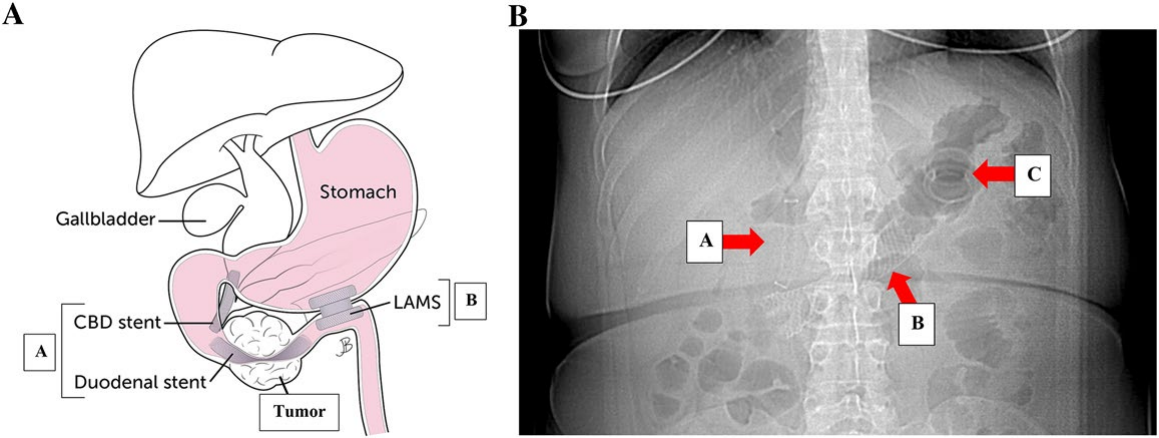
A Location of common bile duct (CBD), duodenal, and GJ stents relative to duodenal mass. A Cannulation of the bile duct, biliary sphincterotomy, and 8 mm × 60 mm fully covered self-expanding metal stent (FCSEMS, Viabil Fore) placement into biliary duct with distal end in duodenum were performed via ERCP. Circumferential, fungating, obstructing mass in the distal descending duodenum involving the major papilla causing 3 cm stricture was traversed with pediatric colonoscope under endoscopic and fluoroscopic guidance. A 22 mm × 90 mm uncovered self-expanding metal stent (USEMS, WallFlex BSCI) was placed across the duodenal stricture, with the proximal end at the level of the major papilla near the previously placed biliary stent. B LAMS was placed two and a half months later. B Abdominal X-ray with red arrows pointing to CBD (A), duodenal (B), and LAM (C) stents
